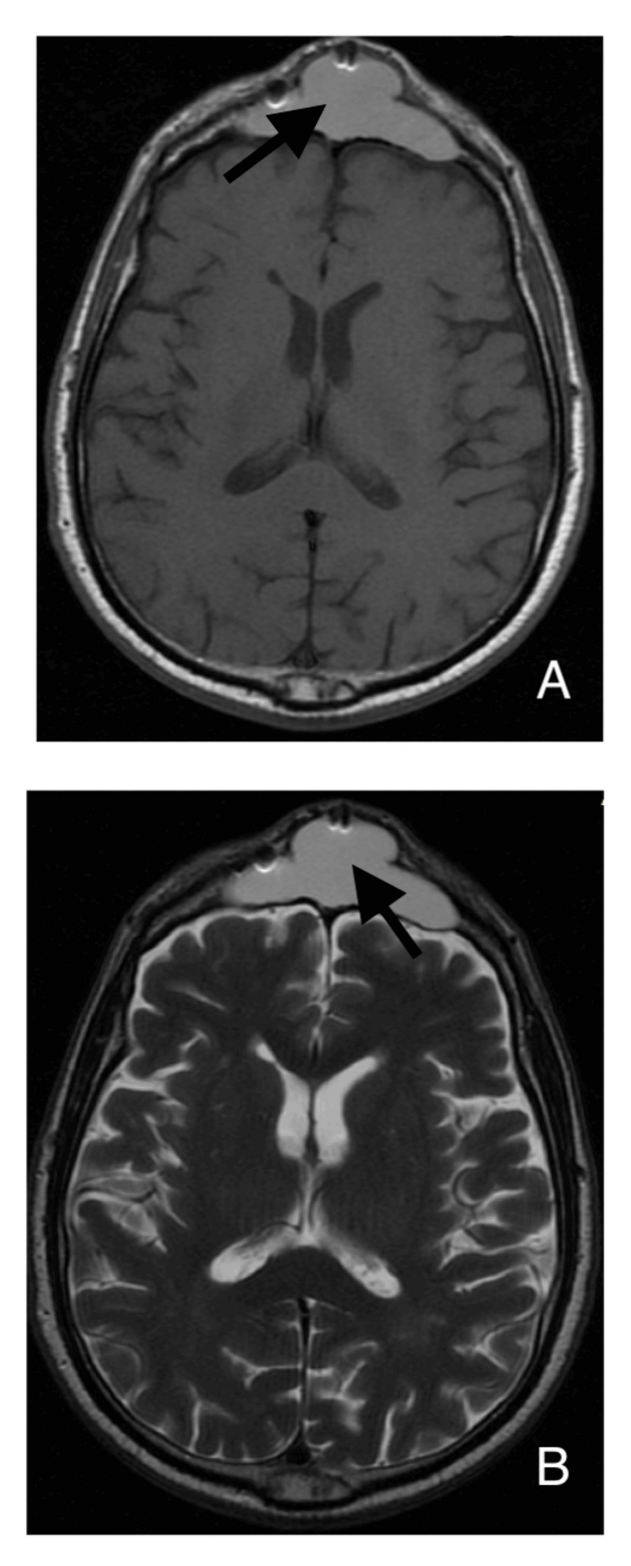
T1 and T2 axial MRI scan showing a hyperdense frontal lesion. MRI: magnetic resonance imaging
